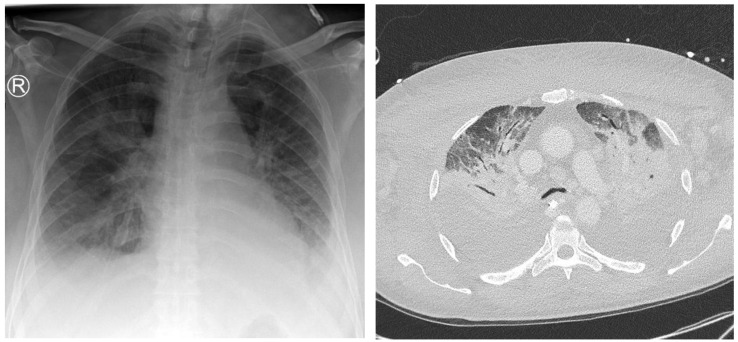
A 75-year-old patient with a focus of unknown origin and uncertain signs of pneumonia on preliminary radiography. Left: Radiograph showing bilateral opacities in the central and inferior fields. Right: CT scan showing extensive bilateral pulmonary infiltrate and pleural effusion.
